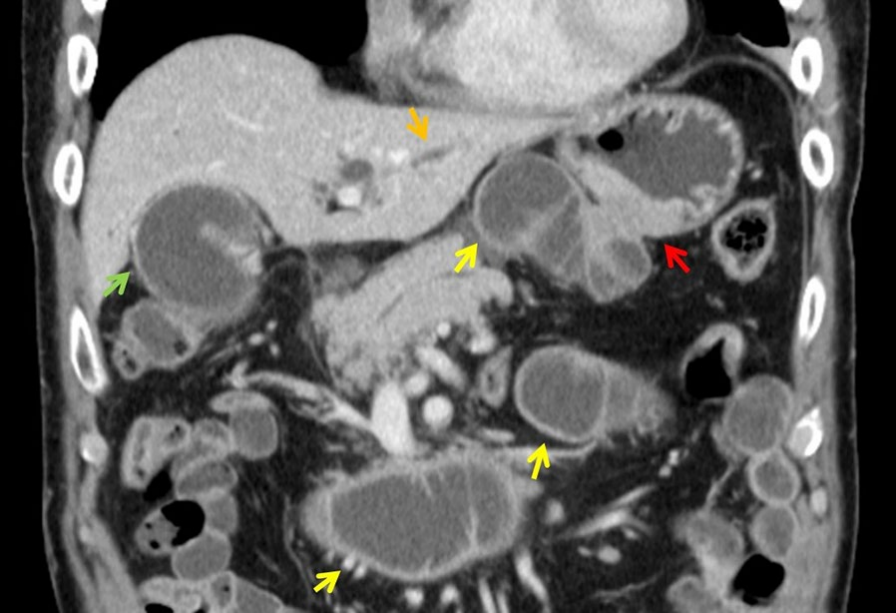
Abdominal contrast-enhanced computed tomography at initial presentation. The anastomosis site of the gastrojejunostomy (red arrow) was thickened. In addition to the afferent loop (yellow arrows), the common bile duct, bilateral intrahepatic bile ducts (orange arrow), and gallbladder (green arrow) were also significantly dilated
